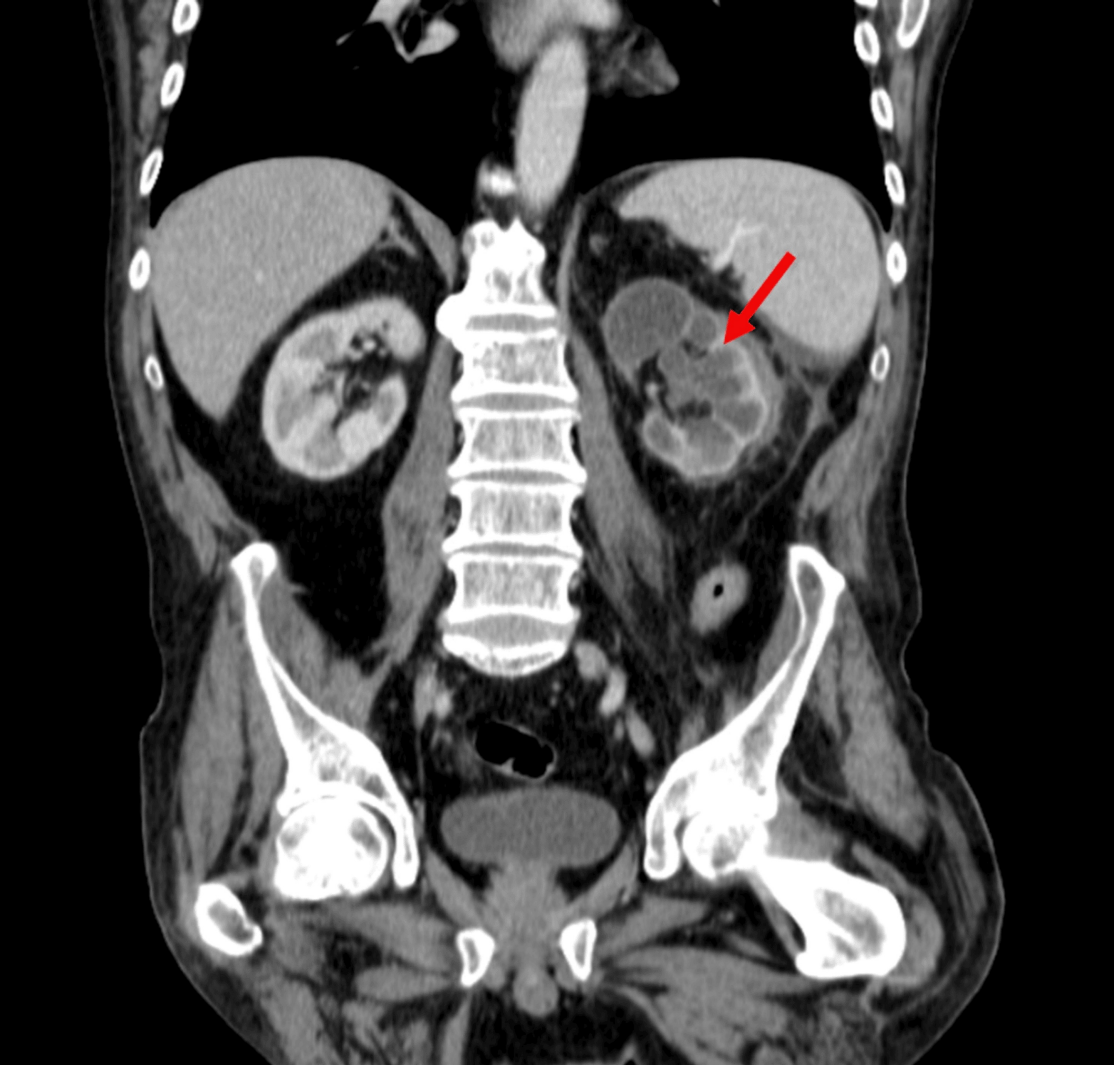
Control abdominal CT scan showing slight posterior left peri-renal densification, adjacent to the drain, with a slight reduction compared to the previous examination (measuring 47 x 26 mm).
